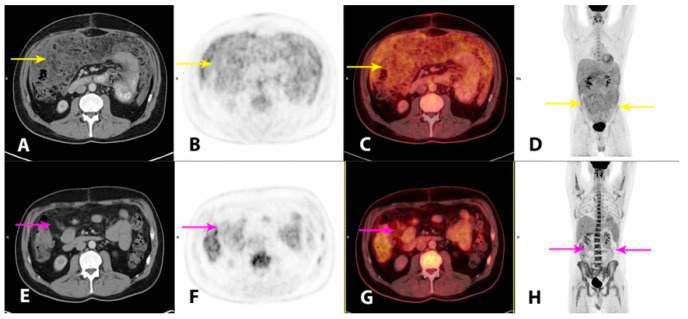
Hybrid imaging with 2-deoxy-2-[^18^F]fluoro-D-glucose positron emission tomography/CT (2-[^18^F]FDG PET/CT) was performed for the staging of the disease and as baseline for monitoring of the treatment response. Imaging performed 1 h post-injection of 4.0 MBq/kg 2-[^18^F]FDG showed a moderately metabolically active, irregular thickening of the peritoneal folds and extensive carcinomatosis-like infiltration in the omentum, mesentery and the pelvic cavity (A–D, yellow arrows). There was minor ascitic fluid in the abdominal and pelvic cavities. There were also metabolically active, enlarged lymph nodes in the mediastinum and upper retroperitoneum. The findings were considered highly suspicious for malignancy. At this point, plasma lactate dehydrogenase (LDH) levels, a future tumor marker in this patient, were elevated (366 U/L; reference range 105–205 U/L). An initial steroid treatment with prednisolone 100 mg/daily had a good effect on the tendency of the recollection of ascites. However, as no standardized treatment for this patient category without comorbidity exists, a hematological specialized tumor board, after consent from the patient, decided to administer a chemotherapy regimen of ifosfamide, carboplatin and etoposide (ICE), which was initiated one month after initial hospitalization. The patient tolerated the treatment well. At clinical follow-up after two cycles of ICE, the patient’s wellbeing was improved, the plasma LDH was normalized and imaging with 2-[^18^F]FDG PET/CT demonstrated a substantial partial response (PR), both metabolically and morphologically, of the previously described findings in the peritoneum. There were still metabolically active, carcinomatosis-like findings in the omentum, mesentery and on the liver surface (E–H, magenta arrows). There were no pathological lymph nodes. After completion of four cycles of ICE, the metabolic complete response (CR) and a further morphological PR were reported on follow-up 2-[^18^F]FDG PET/CT (not shown). The plasma LDH was still within a normal range. The patient tolerated the fifth and final cycle of ICE well.
